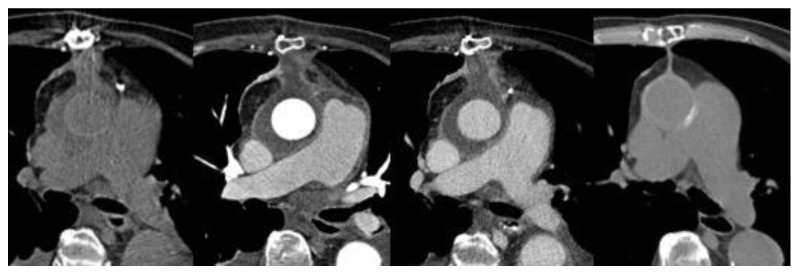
Post-surgical ascending aortic repair (Bentall procedure) 1-month CT scan. From left panel to right: unenhanced CT, arterial phase and late phase CT images show aortic graft patency with perigraft fluid and stranding. These findings can be considered a typical post-operative appearance as confirmed by their disappearance in the 3-month unenhanced CT image (right image).
